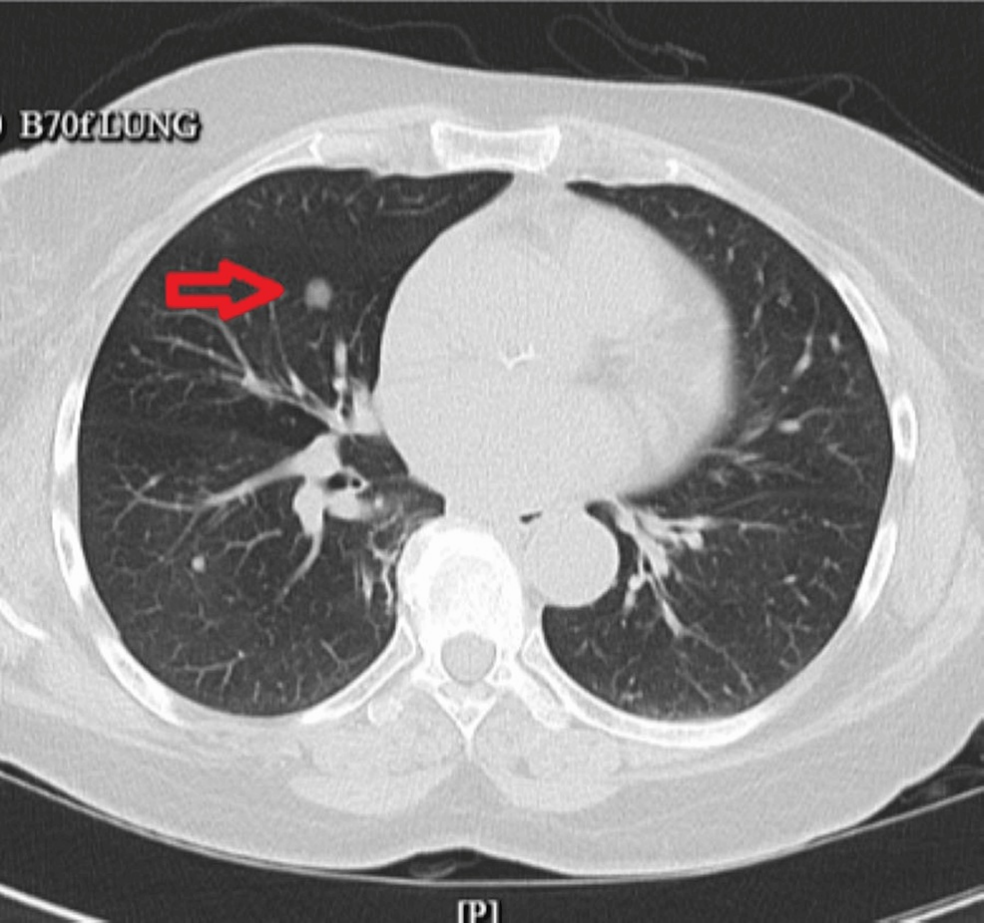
CT of the chest showing a dominant 10 mm pulmonary nodule
